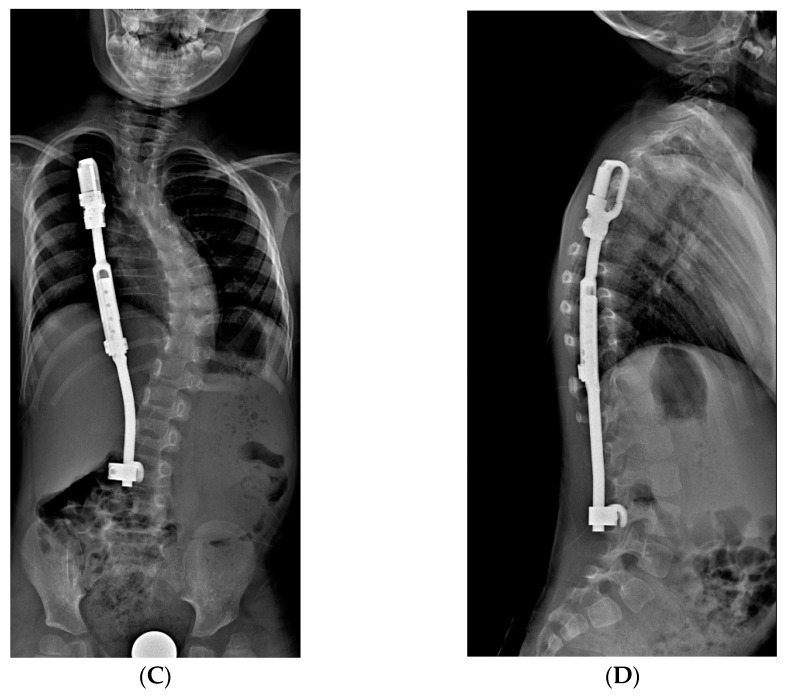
X-rays of early onset scoliosis (EOS) corrected with vertical expandable prosthetic titanium rib (VEPTR): (A) pre-operative antero-posterior X-ray view; (B) post-operative antero-posterior X-ray view; (C) pre-operative lateral X-ray view; (D) post-operative lateral X-ray view.
